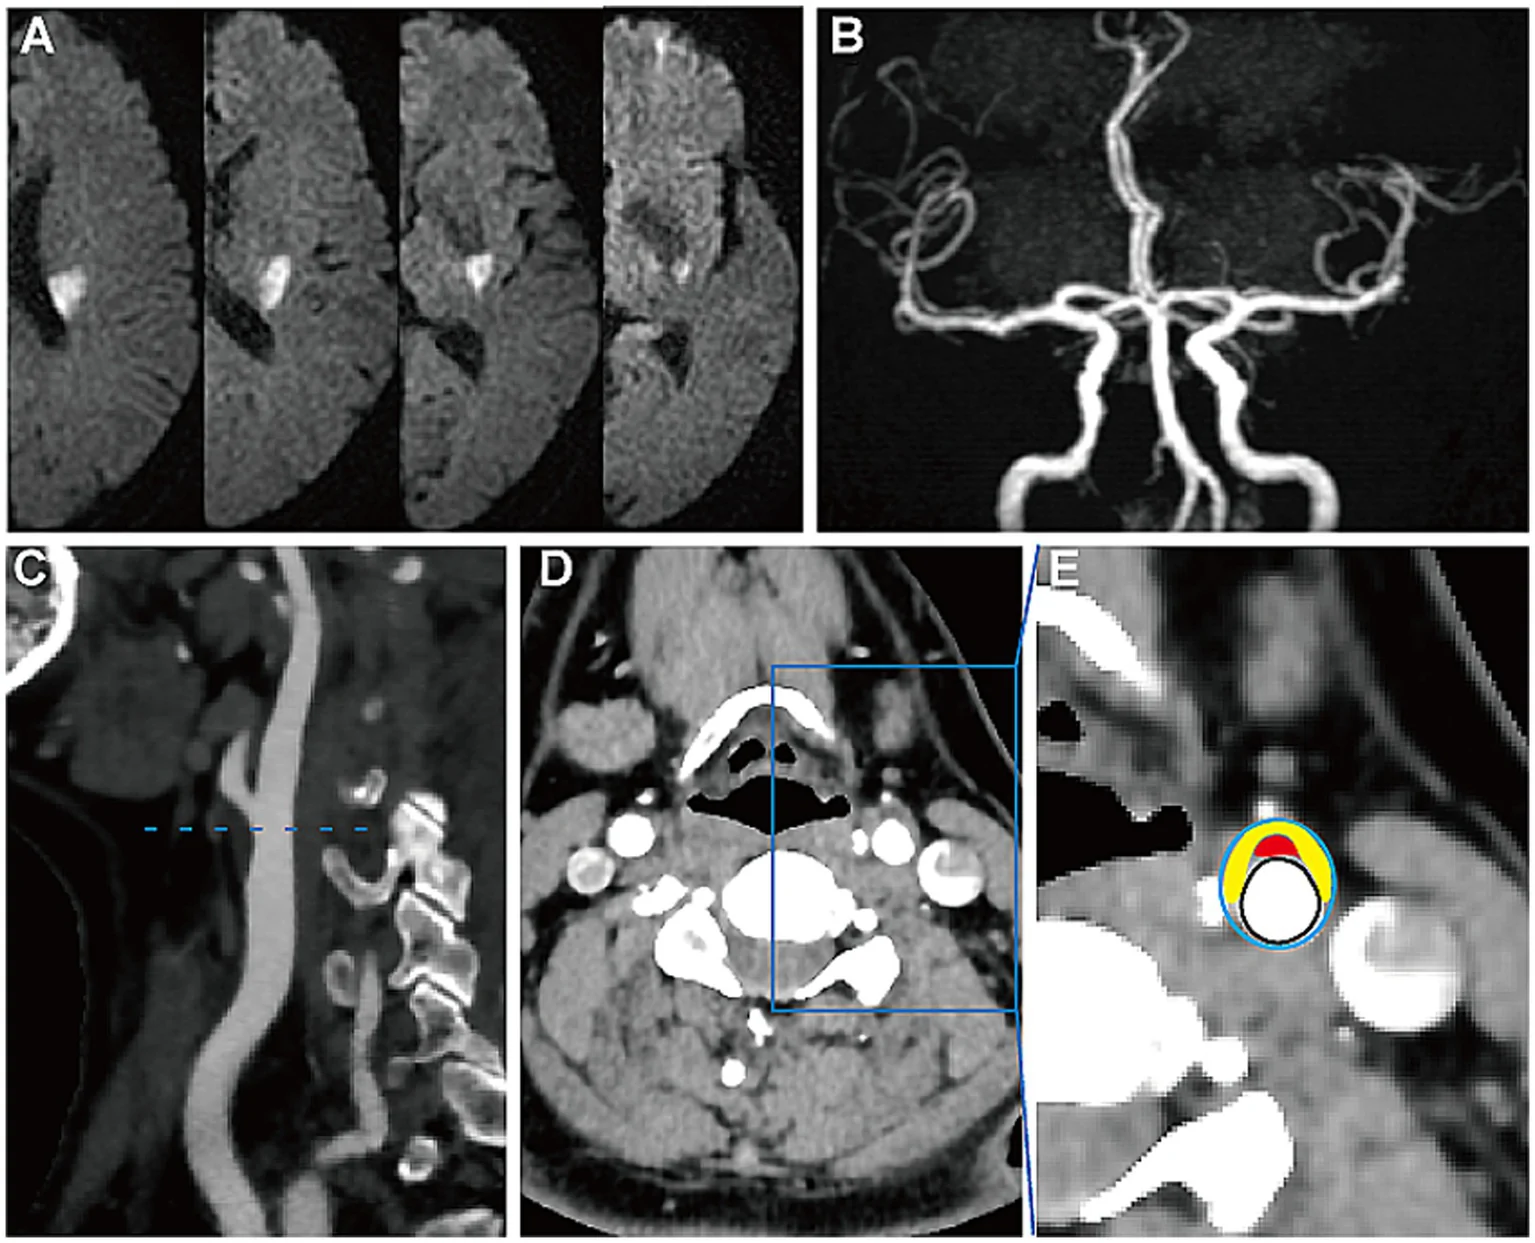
Representative imaging findings in a patient with LSA-territory BAD. (A) Diffusion-weighted imaging demonstrates an acute infarction within the LSA territory. (B) Magnetic resonance angiography shows no significant stenosis in the ipsilateral parent artery of the responsible vessel. (C) The reconstructed image reveals mild stenosis of the Left Common carotid artery. (D,E) Computed tomography angiography identifies a NRS plaque in the Left Common crotid artery. The low-attenuation core (red) is surrounded by a higher-attenuation rim (yellow), while the contrast-enhanced lumen appears white. LSA, lenticulostriate artery; BAD, branch atheromatous disease; NRS, napkin-ring sign.
